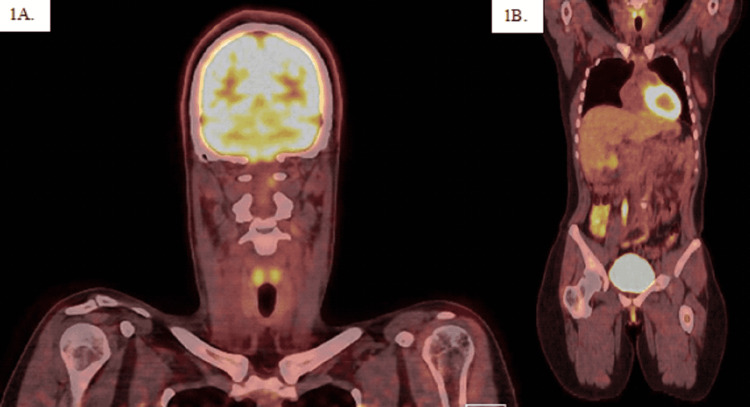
Rosai-Dorfman disease involving the left C1-C2 nerve root Positron emission tomography-computed tomography showed mild hypermetabolic activity within a nodular soft tissue density at the left C1-C2 level (A.) without systemic involvement (B.)
